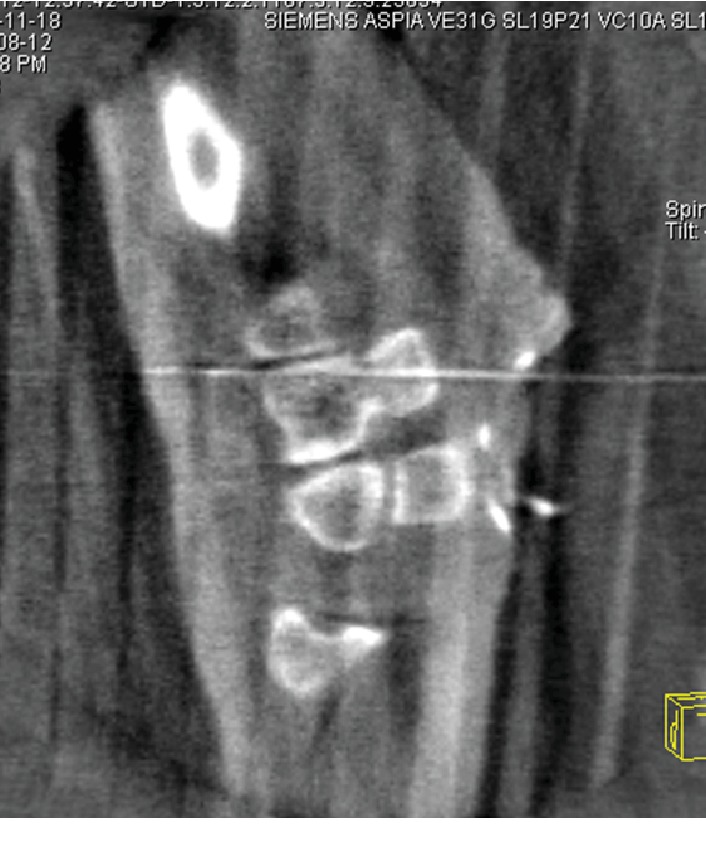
Intraoperative CT scan (sagittal view) showing the anatomical reduction of the hamate hook.
